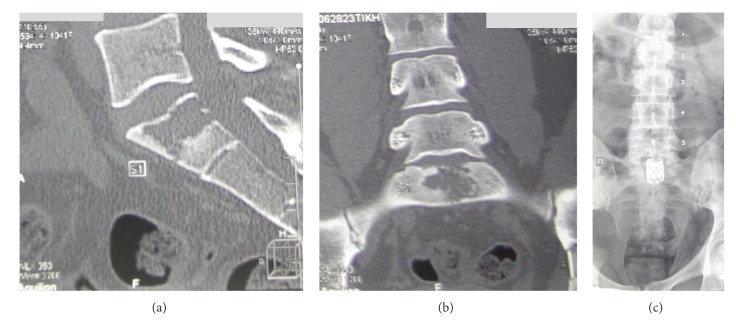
Sagittal (a) and frontal (b) CT showing osteolytic bone lesions in S1, sized 2.9∗2.1∗2.2 cm. (c) Frontal X-rays of lumbosacral region of spine, showing spinal fusion with titanic block.
